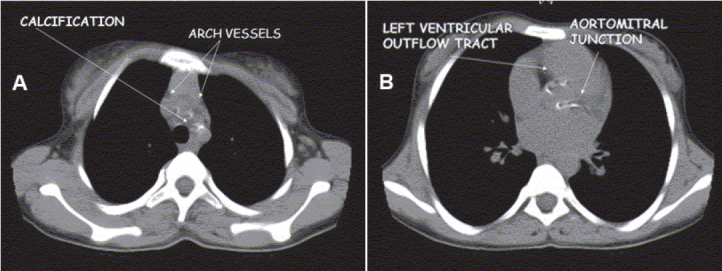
Axial thoracic CT image showing (A) calcifications in the arch vessels, and (B) the aorto mitral area
